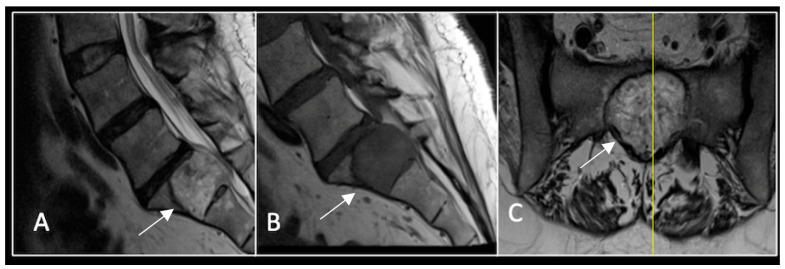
MRI demonstrating a destructive lobular sacral mass (arrow) with invasion of the S1 vertebral body and complete compression of the cauda equina neural elements. The yellow line on the axial image represents the plane of the corresponding axial section. (A) T2W sagittal; (B) T1W sagittal; (C) T2W axial.
